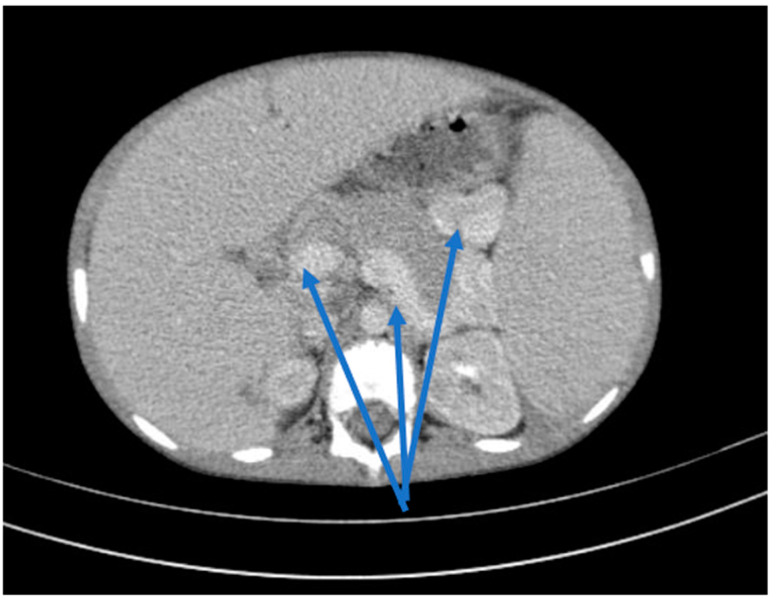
Axial CT scans, bilateral medullary nephrocalcinosis (blue arrow).
